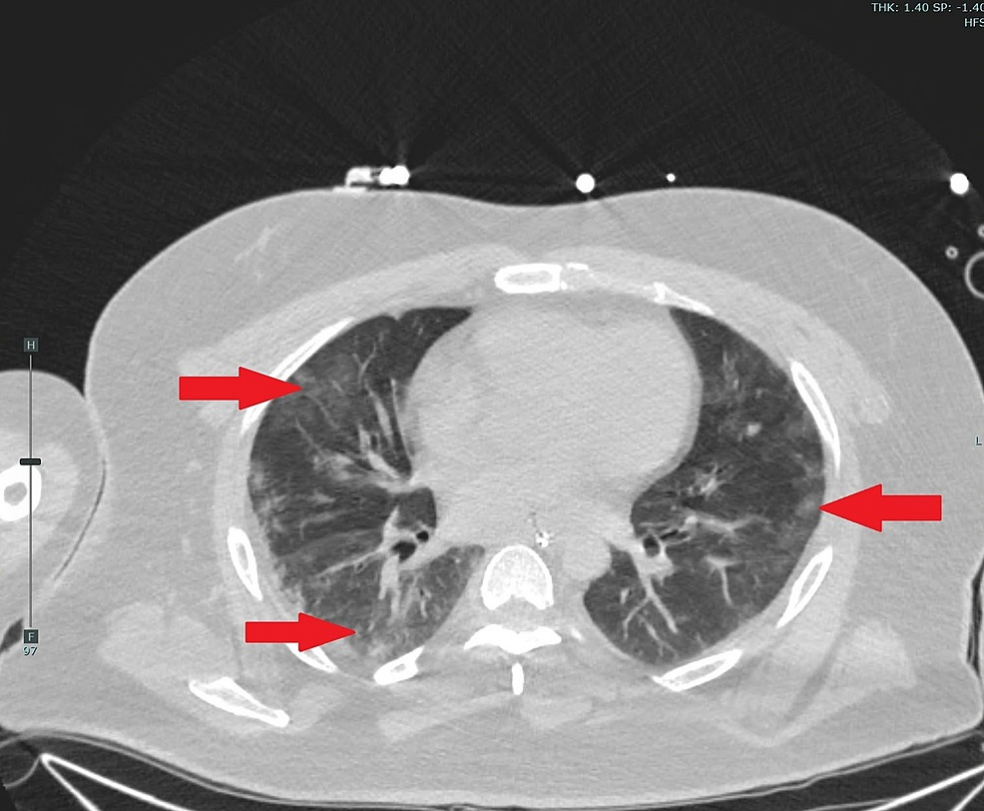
Non-contrast computed tomography chest showing bilateral ground glass opacities (arrows) suggestive of atypical pneumonia.
